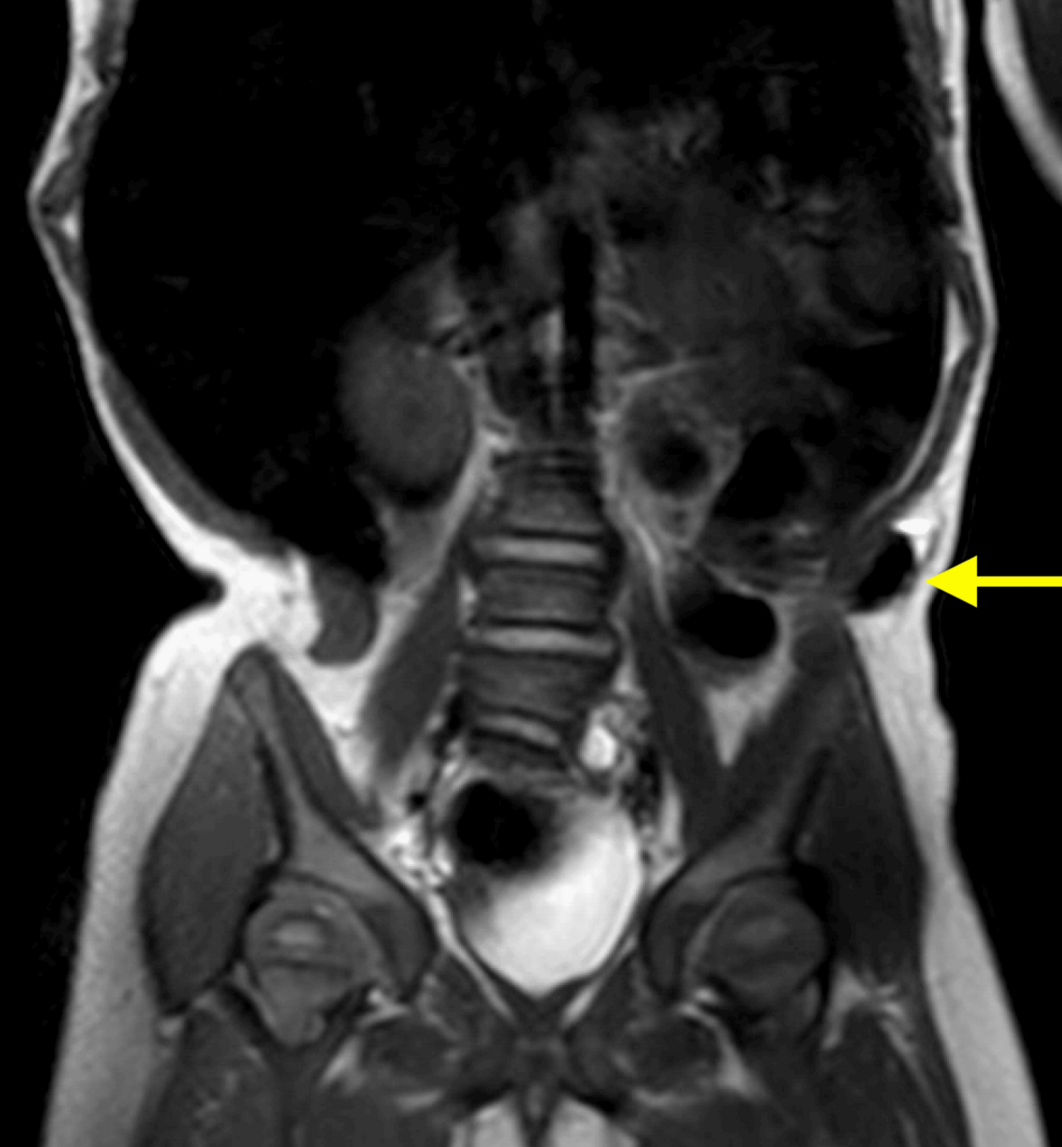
Coronal view of T2-weighted MRI image demonstrating the left lumbar hernia containing bowel.
